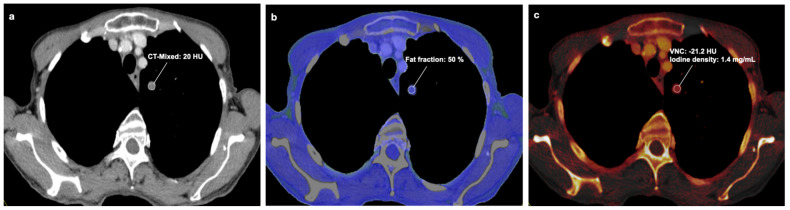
A 72-year-old patient who had undergone portal venous DECT and was found to have a 14 mm pulmonary nodule, which was subsequently diagnosed as a hamartoma. (a) Linearly blended DECT images are shown (equivalent to single-energy CT) with a CT mixed value of 20 HU. (b) The corresponding fat map in the same slice position shows a fat percentage of 50%. (c) The iodine mapping shows a ROI in the same slice position with a VNC of −21.2 HU and an iodine density of 1.4 mg/mL.
